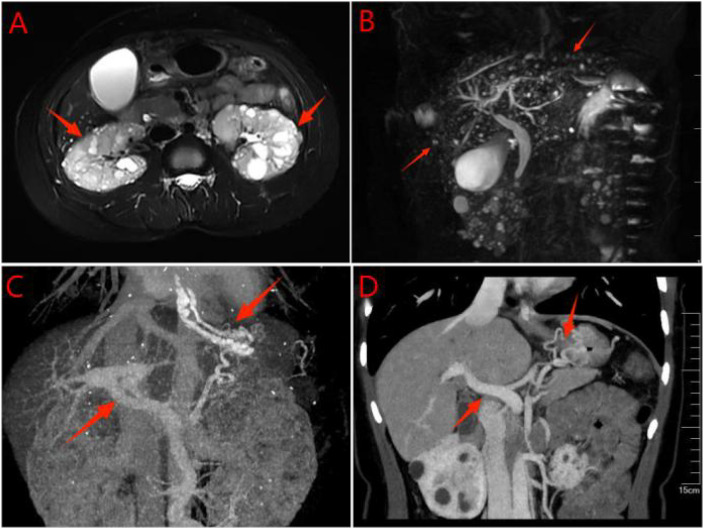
(A) Upper abdomen enhanced MRI (axial view, T2WI). The red arrows point to bilateral renal multiple nodular hyperintense lesions, confirming the feature of ADPKD. (B) Upper abdomen enhanced MRI (coronal view). The red arrows indicate intrahepatic cystic lesions presenting typical “starry sky” pattern without communication with the biliary tract, consistent with VMC. (C, D) Portal vein CTV ((C) coronal maximum intensity projection; (D) sagittal reformation). The red arrows mark dilated portal trunk (diameter ~17.5 mm) and obvious esophagogastric fundal varices caused by portal hypertension respectively.
